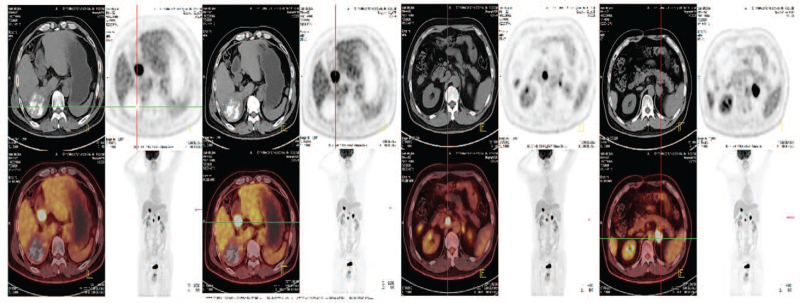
PET-CT showed the FDG of the left adrenal metastasis, hilar, and retroperitoneal enlargement lymph nodes were increased FDG, but there was no FDG intake in the liver and inferior vena cava area. FDG = fluorodeoxyglucose, PET-CT = positron emission tomography-CT.
